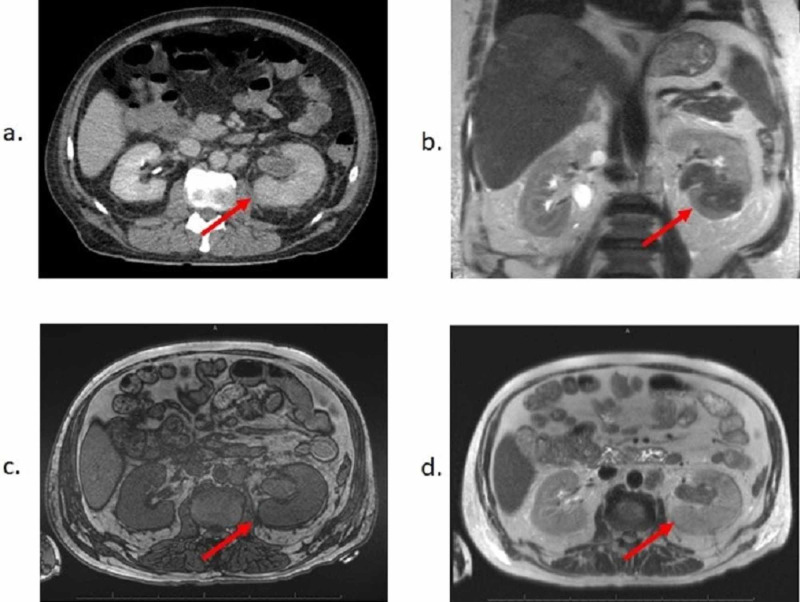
Initial Imaging of Renal Pelvis Mass. Representative images from (a) CT pelvis with contrast, (b) MRI pelvis scout, (c) T1-weighted MRI, and (d) T2-weighted MRI demonstrating lesion occupying left renal pelvis with decreased enhancement and delayed excretion of contrast, with adjacent soft tissue stranding. Mass in left kidney is restricting and extends into the renal parenchyma into the lower pole of left kidney, measuring up to 7 cm in greatest diameter.
